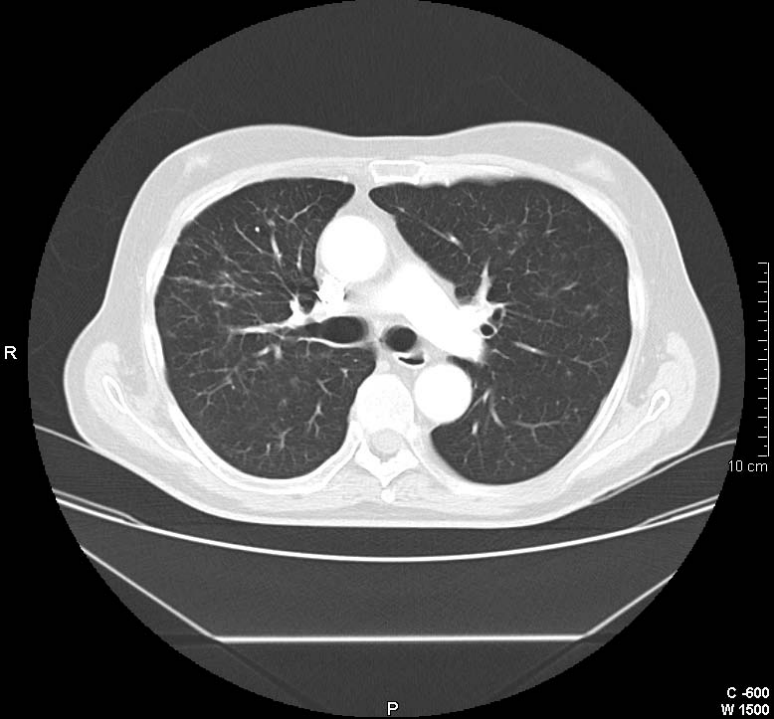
(Three months later) a CT scan shows almost complete resolution with minimal residual reticular opacities in the right lung.
